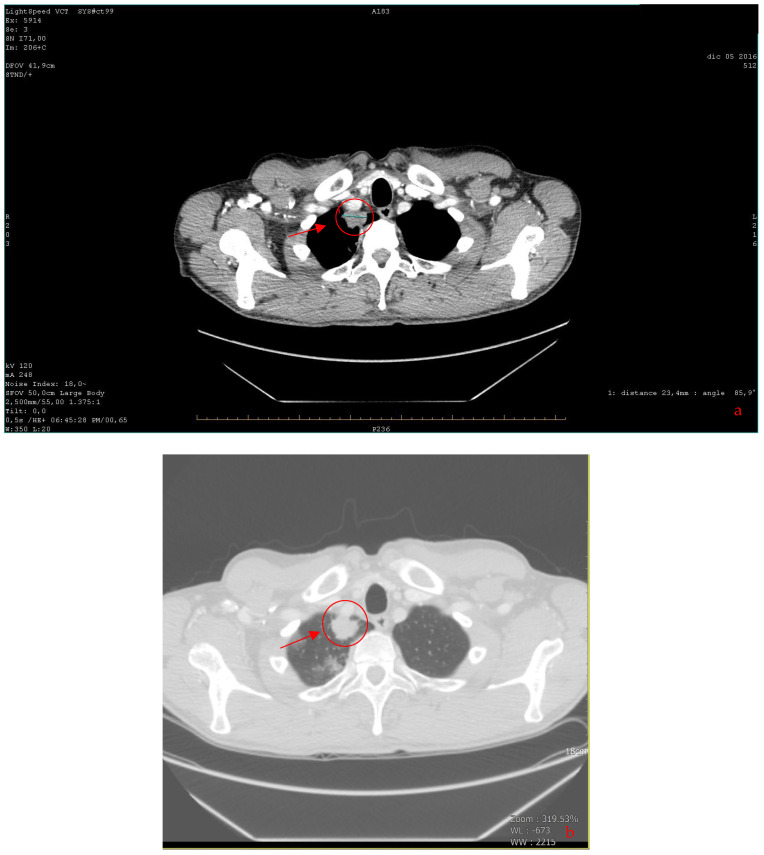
(a,b) The axial CT scan revealed a solid, nodular-like, expansive process in the right upper lobe of the lung (marked with a red circle) (a). To the right: lung windowing (b) (2016).
